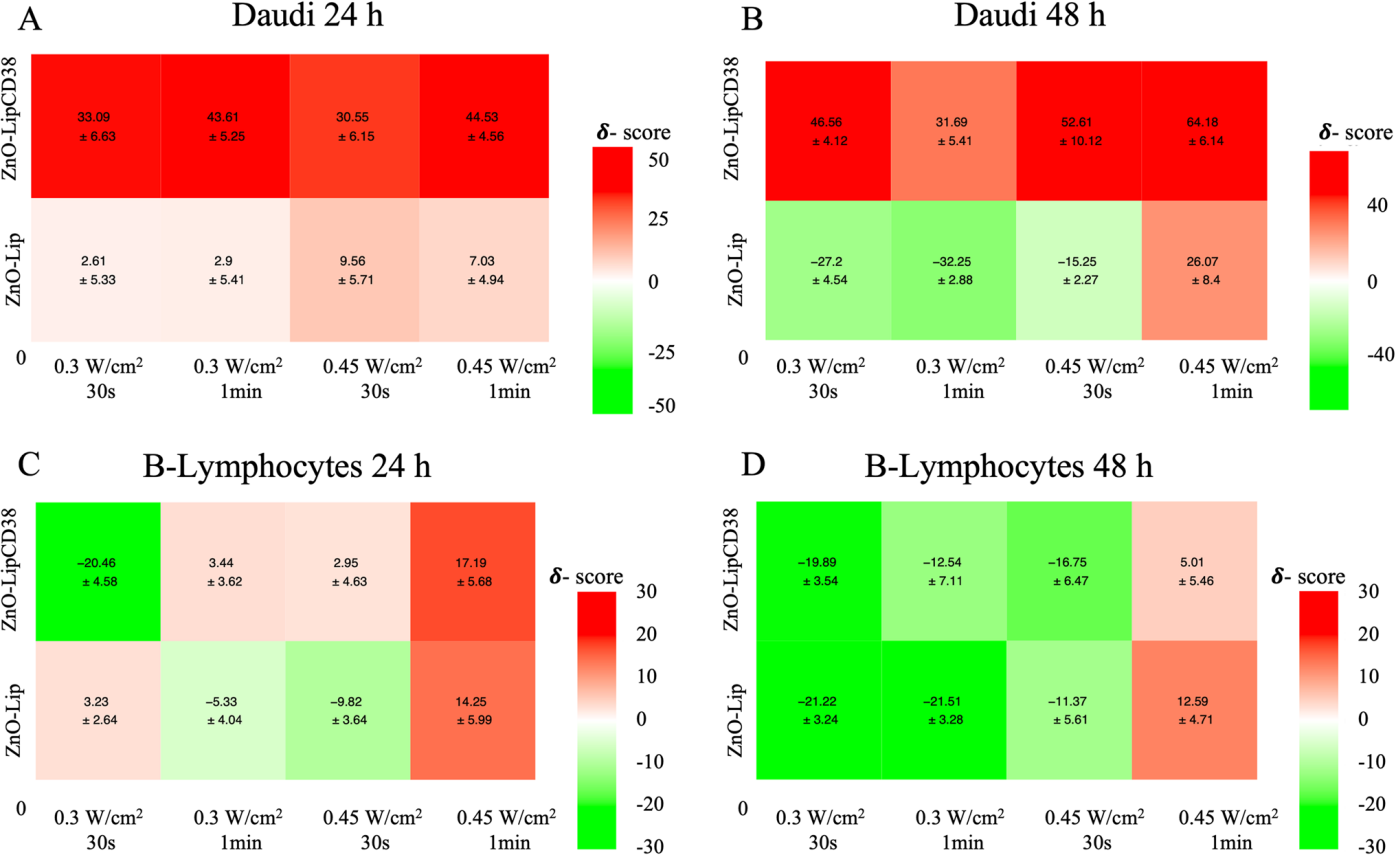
Evaluation of the synergy derived from the combination of ultrasound irradiation and the administration of ZnO-Lip and ZnO-LipCD38 on Daudi, at 24 h (a) and 48 h (b), and on B-Lymphocytes, at 24 h (c) and 48 h (d). The effects generated by the administration of the two treatments (US and ZnO-Lip/ZnO-LipCD38) can be evaluated by the synergy score (δ); it can be < − 10 and it represents antagonistic interaction, > − 10 and < 10 and it represents additive interaction, or it can be > 10 and it represents synergistic interaction. The heatmaps highlight synergistic and antagonistic dose regions in red and green colors, respectively. Cells were previously incubated with 40 μg/mL of nanoconstructs 24 h before the US treatment The analysed data were at least in triplicate
